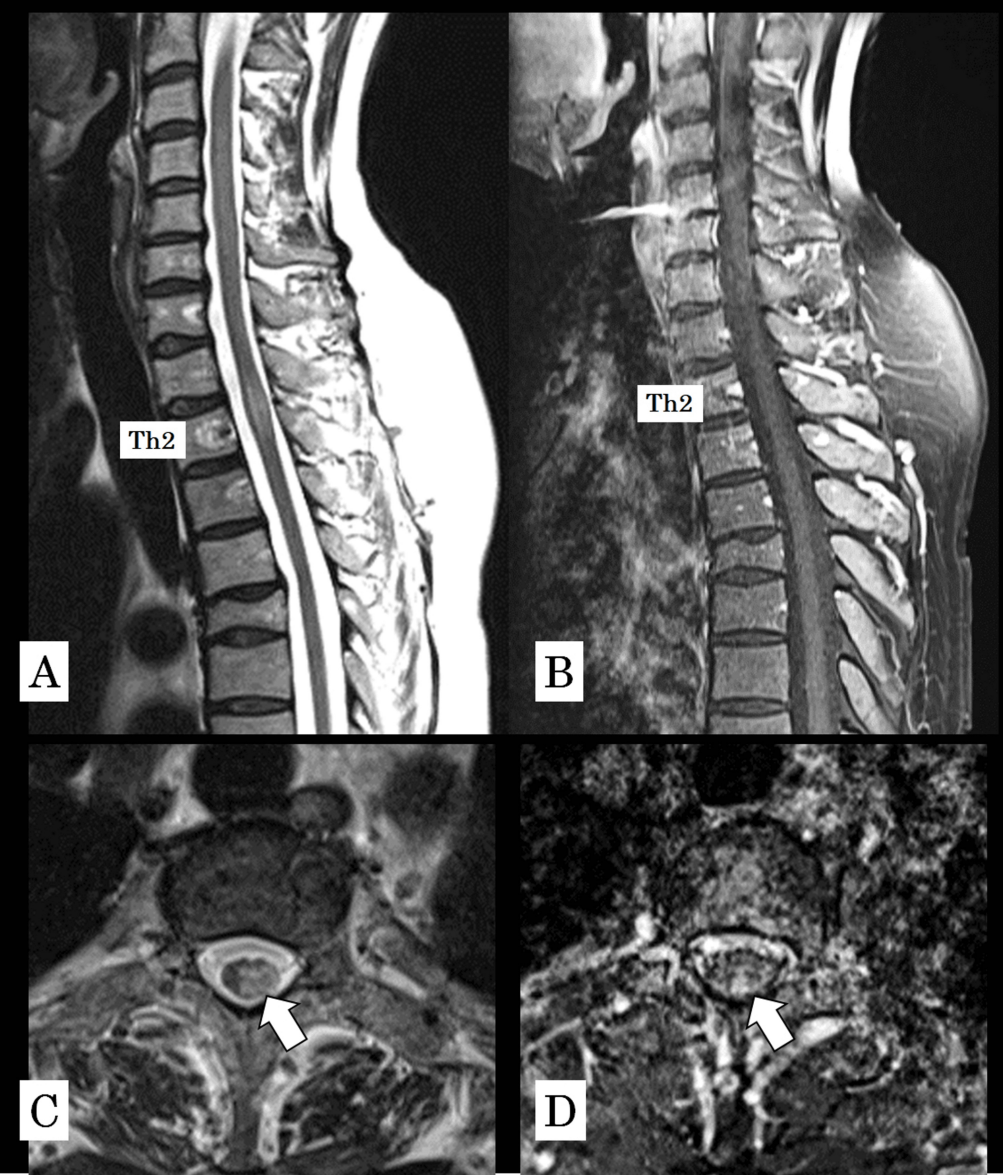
Thoracic spine MRI on day 7 of treatment. (A) T2-weighted sagittal image showing improvement in T2 hyperintensity and reduction in lesion size. (B) Gadolinium-enhanced sagittal image demonstrating decreased contrast enhancement compared to initial imaging. (C) Axial T2-weighted image and (D) axial gadolinium-enhanced image confirming reduction in lesion extent (arrows indicate the lesion).
